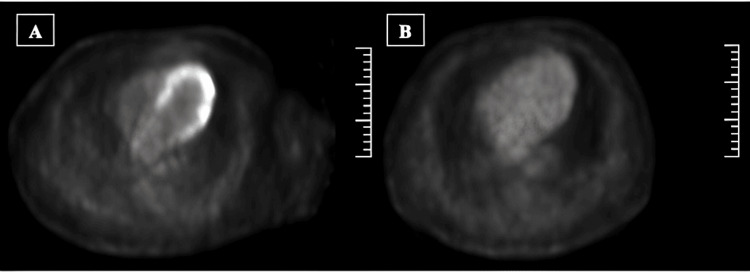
A: 18F-PET-CT with radiotracer uptake in LV walls in the lateral and posterior region. B: Control 18F-PET-CT with no uptake of the radiotracer after two years of starting treatment. 18F-PET-CT: fluor-18 positron emission tomography-computed tomography, LV: left ventricle
